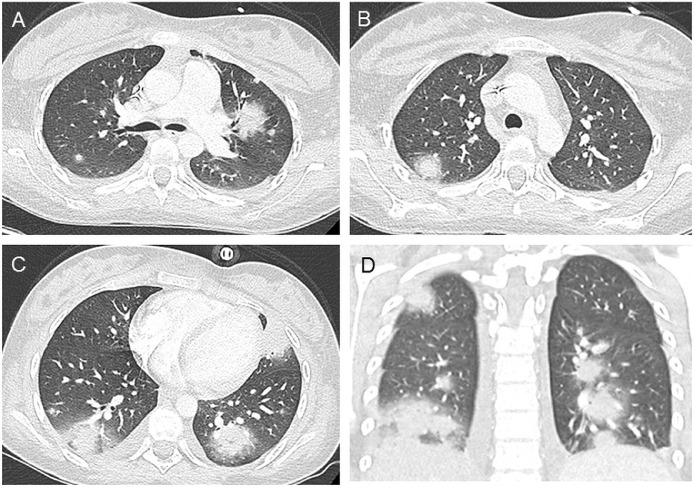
Baseline contrast-enhanced chest computed tomography (CT). Baseline chest CT was performed for persistent febrile medullary aplasia despite the administration of piperacillin/tazobactam and amikacin, followed by ciprofloxacin and linezolide. Axial (A, B, C) and coronal (D) slices show bilateral nodular opacities of mixed topography (central and subpleural) surrounded by halos of ground-glass opacities. Some lesions began to be excavated. These patterns were highly suggestive of invasive pulmonary aspergillosis.
